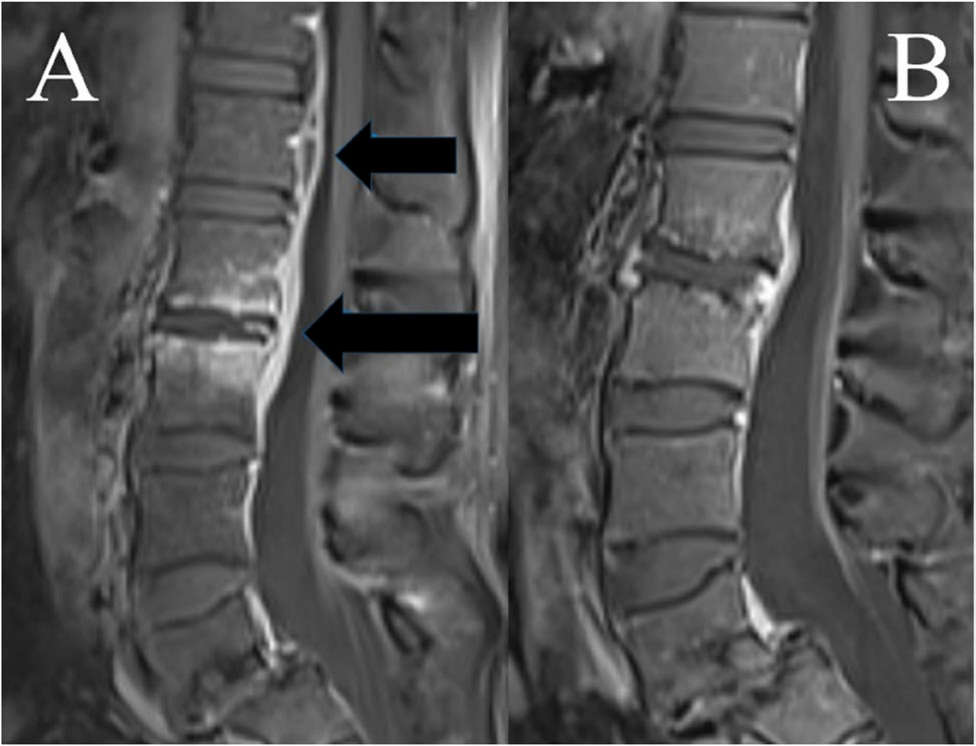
A MRI of the lumbar spine during the first hospitalization, showing edema in the L2-L3 intervertebral disc and contrast-enhancement in the endplates of L2-L3 indicating spondylodiscitis. Dorsal to L1, an epidural abscess can be seen (30 × 6 mm). B MRI of the lumbar spine 8 months after hospital discharge showing regression of the epidural abscess as well as partial regression of the contrast-enhancement in the paravertebral and prevertebral structures of L2-L3
